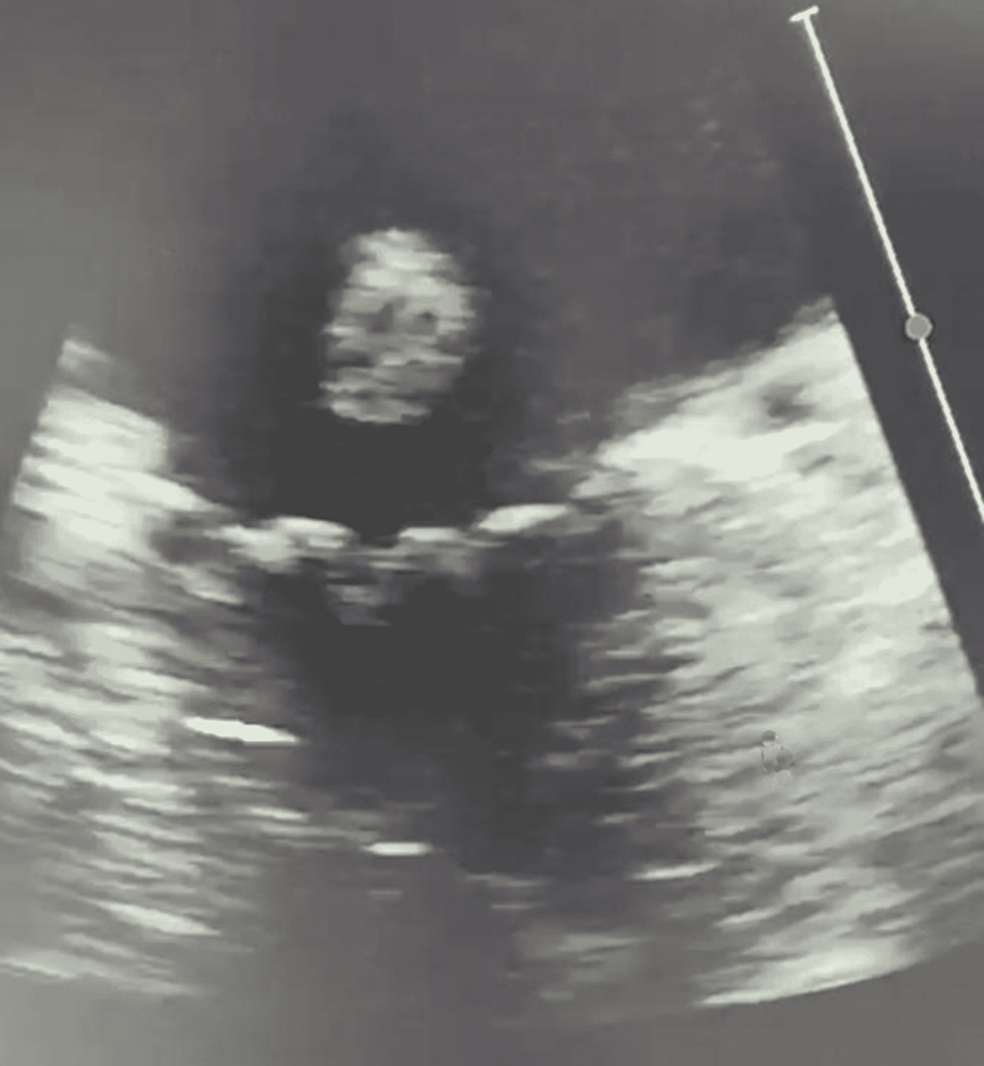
Transoesophageal echocardiogram demonstrating flail anterior mitral leaflet.
